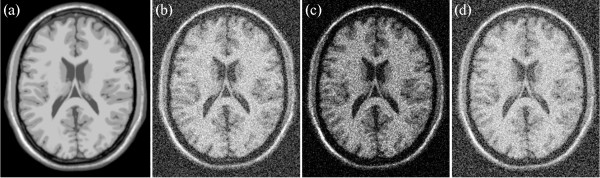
An example of T1 image: (a) original T1 weighted MRI, (b) 17% noisy T1 weighted MRI, (c) squared magnitude of the noisy T1 weighted MRI, (d) the forward variance-stabilization transformation of the noisy T1 weighted MRI.
